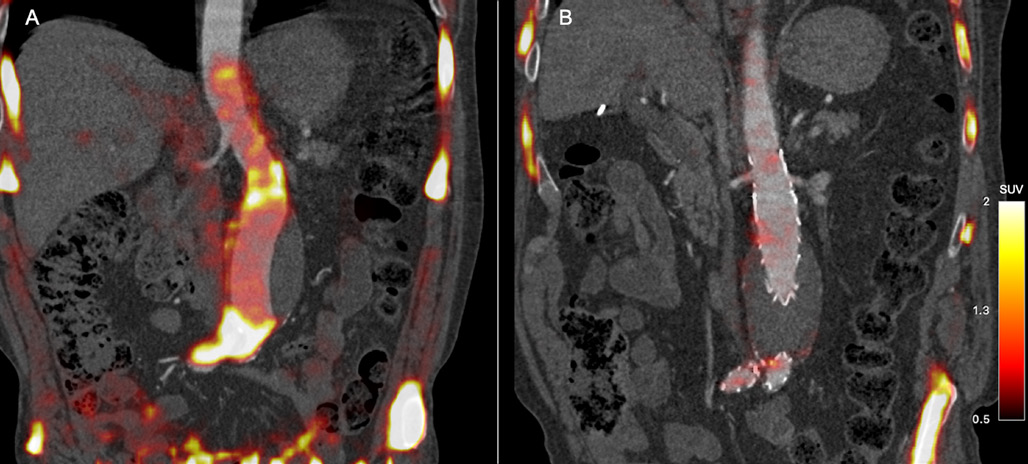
Images from a representative patient with a preoperative and a postoperative sodium [^18^F]fluoride positron emission tomography and CT angiogram. The CT angiogram is performed immediately after acquisition of PET data. A visual reduction in sodium [^18^F]fluoride uptake can be observed when comparing the preoperative PET-CT (Panel A) and the postoperative PET-CT (Panel B) in the same patient. SUVs represented in the colour scale bar. PET, positron emission tomography; SUVs, standard uptake values.
